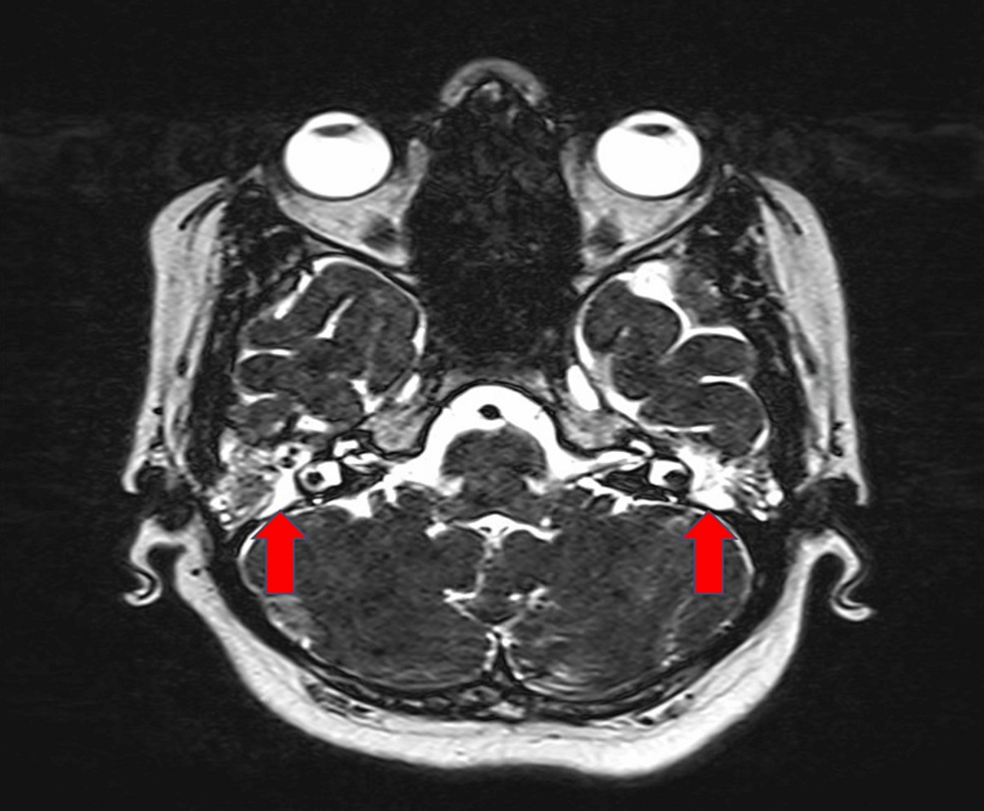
Fluid presence in middle ear on preoperative short-term T2 sequence MRI MRI: magnetic resonance imaging
